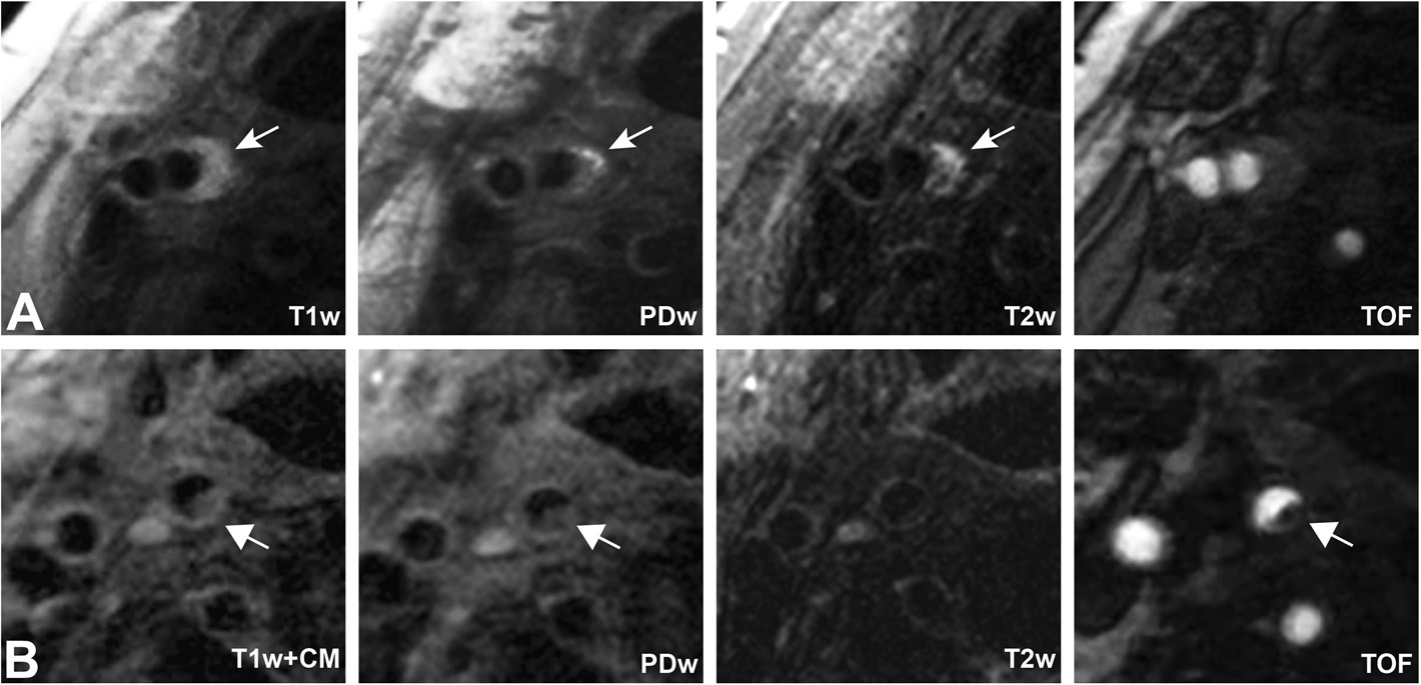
Axial images of specific AHA-LT VI plaque features in a patient with acute ischemic infarct. High-resolution black blood MRI of the carotid arteries demonstrate a large eccentric plaque with <50% stenosis in the right carotid artery, hyperintense on T1w, T2w and PDw sequences consistent with fresh intraplaque hemorrhage (A, arrow). Axial images 0.6 cm above the bifurcation show an area attached to the plaque, which is hypo- to isointense on T1w + CM, PDw and TOF, consistent with a mural thrombus (B, arrow). (T1w: T1-weighted black blood sequence, PDw: PD-weighted sequences, T2w: T2-weighted sequence, TOF: Time-of-flight MR angiography, T1w + CM: T1-weighted black blood sequence after contrast administration).
